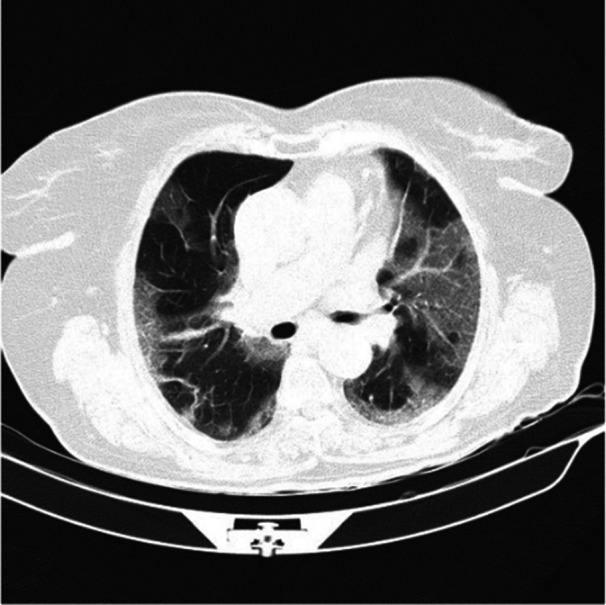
A CT scan section showed severe lung involvement in a 71‐year‐old male/female patient with no history of underlying disease.
